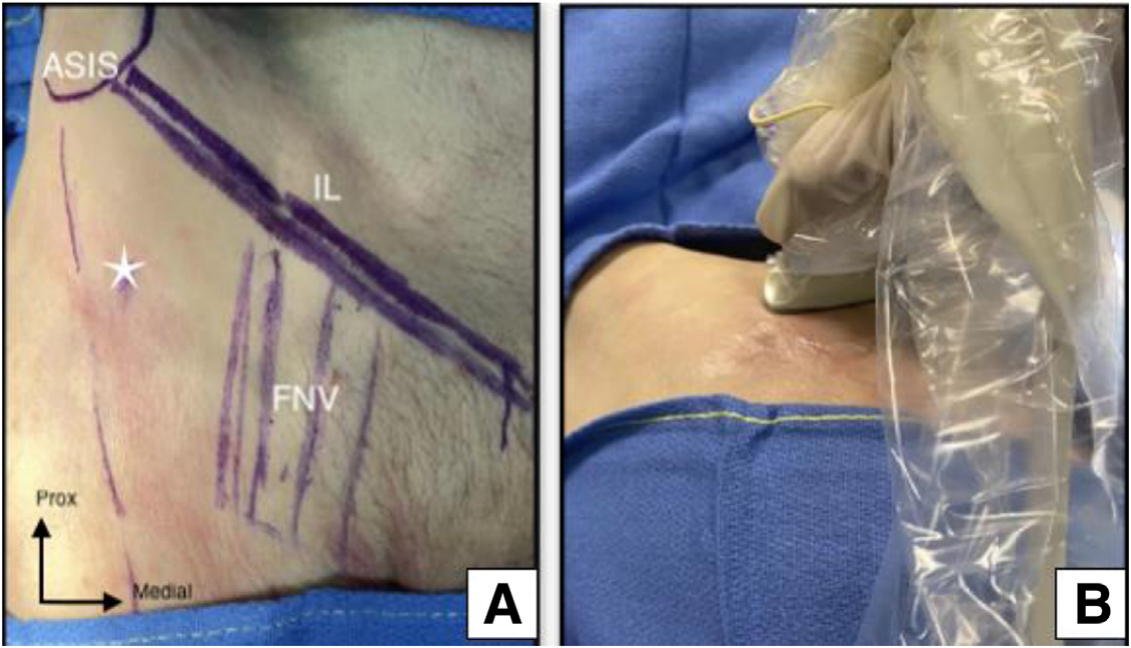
Top view of skin landmarks (A) and ultrasound transducer positioning (B) in left hip and supine cadaveric model. Care should be taken to avoid the nearby femoral neurovascular bundle (FNV). The star indicates the optimal entry point of the needle approach. (ASIS, anterior superior iliac spine; IL, inguinal ligament; Prox, proximal.)
